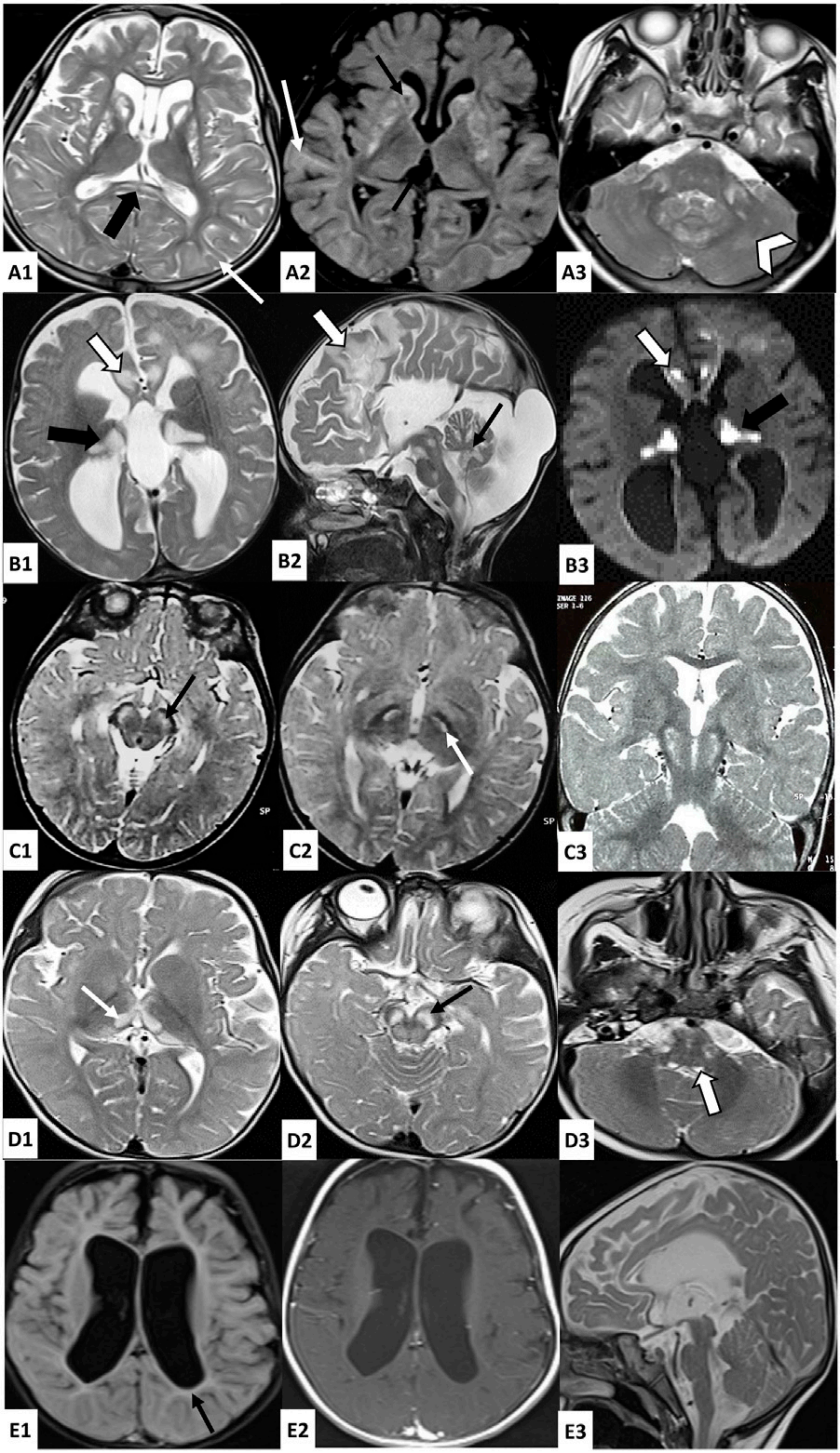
Brain MRI findings in investigated patients. A: Axial T2 (A1, A3) and FLAIR (A2)-weighted images of patient 1 brain MRI showing bilateral and symmetrical striatal hyperintensities, with the presence of cavitations (thin black arrow), associated with T2 and FLAIR hyperintensities of the periventricular and subcortical white matter (thin white arrow), splenium of the corpus callosum (thick black arrow), middle cerebellar peduncles (thick white arrow), and cerebellar white matter (white arrowhead); B: Axial (B1-B3) and sagittal (B2) brain MRI of patient 2 showing bilateral hyperintense lesions in T2 (B1, B2) and diffusion (B3)-weighted images affecting the cortex and subcortical white matter in frontal areas (thick white arrow), the thalami (thick black arrow), the midbrain, the pons, the medulla oblongata, and the cerebellum (thin black arrow), as well as cortico-subcortical atrophy predominant at the subtentorial level and agenesis of the corpus callosum; C: Axial (C1, C2) and coronal (C3) T2-weighted images of patient 3 brain MRI showing bilateral and symmetrical hyperintensities affecting the cerebral peduncles (thin black arrow) and subthalamic nuclei (thin white arrow); D: Axial (D1–D3) T2-weighted images of Patient 4 brain MRI showing bilateral and symmetrical hyperintensities affecting the thalami (thin white arrow), the midbrain (thin black arrow) and the posterior part of the medulla oblongata (thick white arrow); E: Axial (E1, E2) and sagittal (E3) brain MRI of patient 5 showing significant subcortical atrophy with the enlargement of the third and lateral ventricles, mild hyperintensities of the periventricular white matter in FLAIR-weighted image (E1) which are isosignal in T1-weighted image (E2), and hypoplasia of the brainstem.
